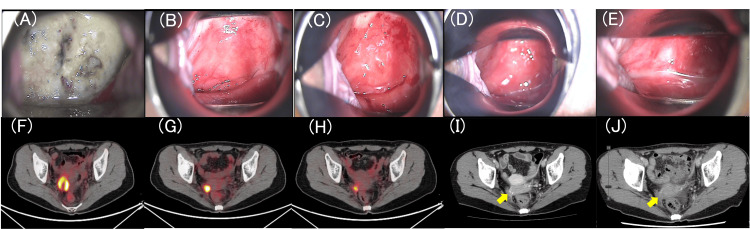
Serial colposcopic and imaging findings during treatment for recurrent cervical cancer. (A) Colposcopy before initiation of chemoimmunotherapy; (B) Colposcopy after six cycles of paclitaxel, carboplatin, and pembrolizumab; (C) Colposcopy after 13 cycles of combination therapy; (D) Colposcopy after 16 cycles (completion of combination therapy); (E) Colposcopy after 35 cycles of pembrolizumab (end of treatment); (F) Axial PET-CT before initiation of chemoimmunotherapy; (G) Axial PET-CT after six cycles; (H) Axial PET-CT after 13 cycles; (I) Axial contrast-enhanced CT after 16 cycles (completion of combination therapy); (J) Axial contrast-enhanced CT after 35 cycles of pembrolizumab (end of treatment). The recurrent/residual lesion is indicated by arrows, where applicable. PET-CT: positron emission tomography-computed tomography
